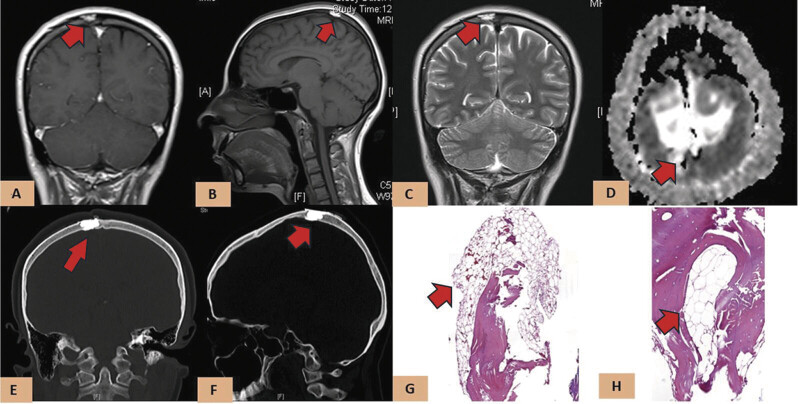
Coronal and sagittal T1-weighted images, as well as a coronal T2-weighted image, reveal a hyperintense lesion compressing the superior sagittal sinus. The lesion measures 26 × 12 × 20 mm. (
